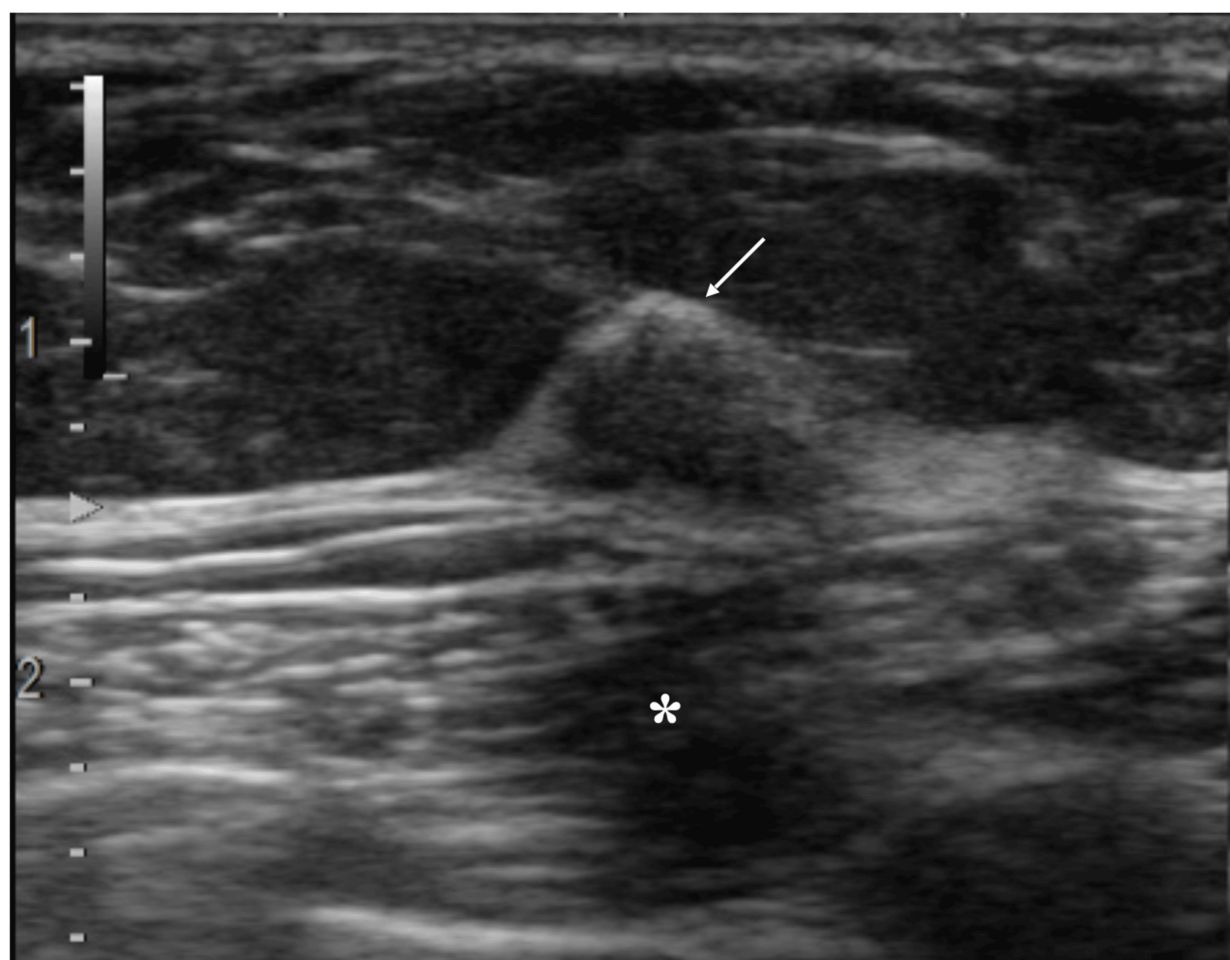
Ultrasound showing a triangular mass with somewhat unclear borders; central part of the anterior borders had very high echogenicity (arrow), while the lateral parts had less echogenicity. The mass had multiple punctate echogenic foci and slightly attenuated posterior echoes (asterisk), strongly suggesting some kind of papillary structures and widespread collagen fibers in the tumor.
